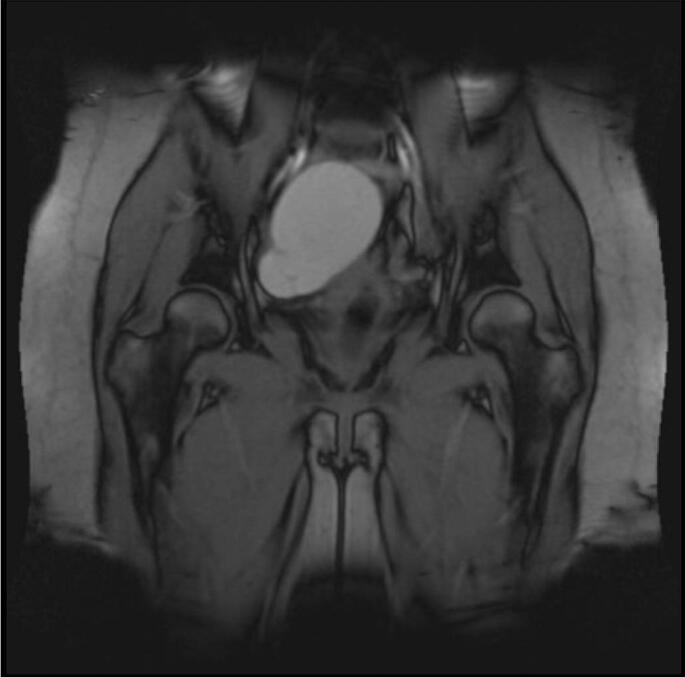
Follow up MRI recommended following increased size of the ovarian cyst which showed the cyst had homogenous hyperintense signal consistent with an endometrioma.
